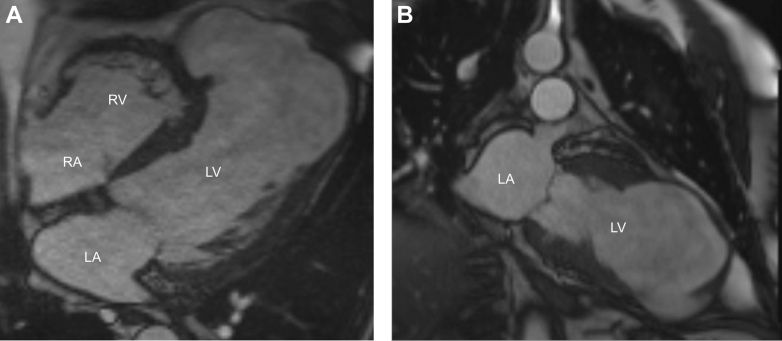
Cardiac Magnetic Resonance Imaging of Left Ventricular Pseudoaneurysm Cardiac magnetic resonance imaging demonstrating a large left ventricular pseudoaneurysm on (A) coronal and (B) sagittal views. The coronal image demonstrates right ventricular collapse due to mass effect from the pseudoaneurysm. LA = left atrium; LV = left ventricle; RA = right atrium; RV = right ventricle.
